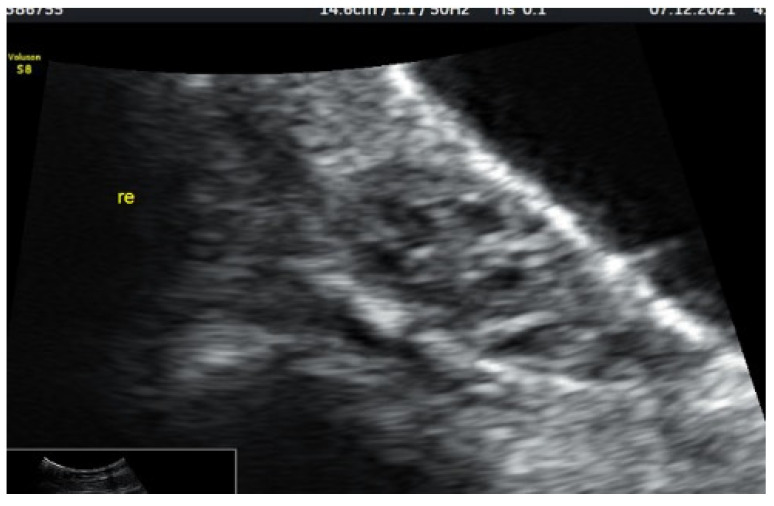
Abdominal sonography of an right (re) ovary in an adolescent girl (photo by the authors).
